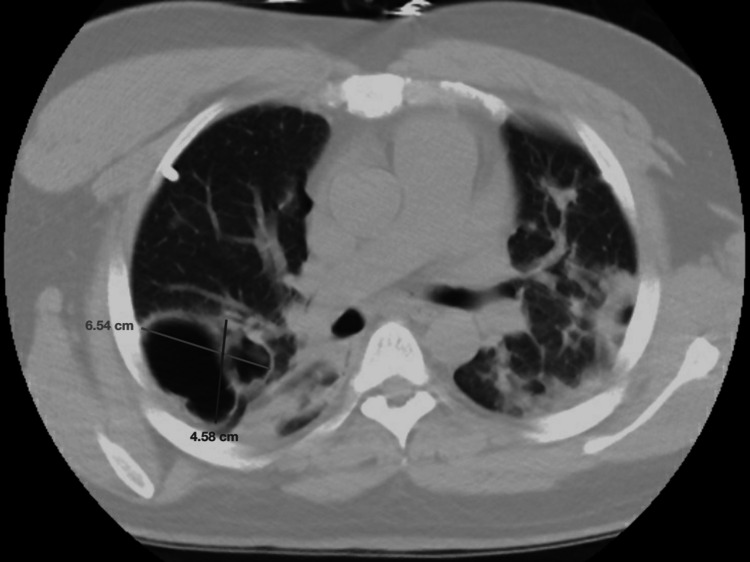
Computed tomography of the chest without contrast demonstrating loculated, air-filled, thick-walled cysts along the right major fissure measuring 6.5 x 4.6 x 3.0 cm.
